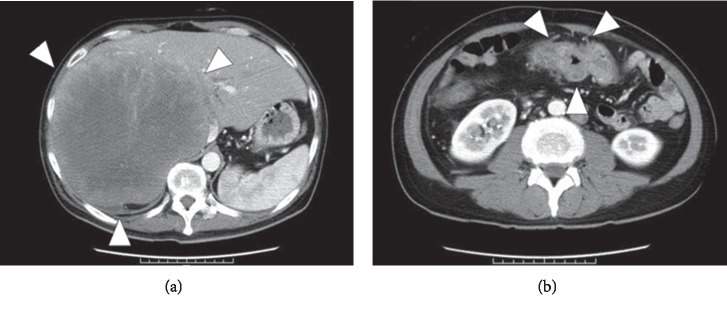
Contrast-enhanced CT scan to determine the primary site of neuroendocrine carcinoma of the thyroid. Tumors were found in the liver (A) and transverse colon (B).
